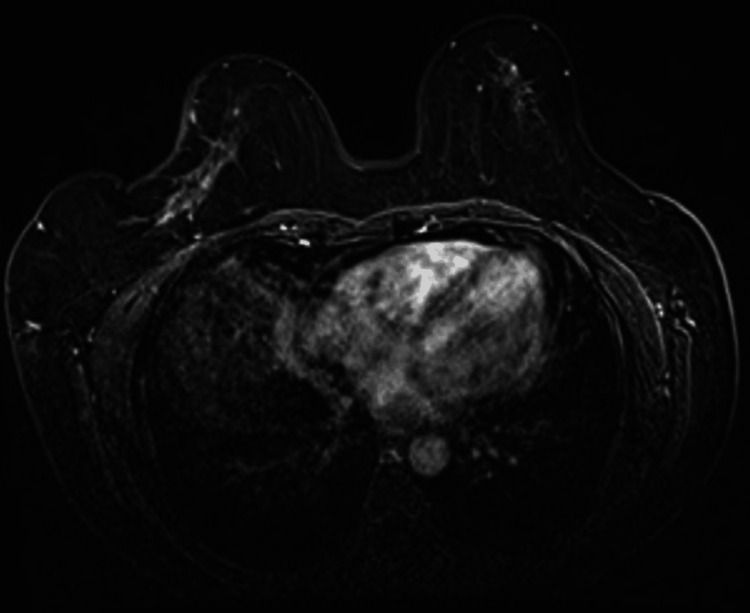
Magnetic resonance mammography (contrast, T1-weighted fat suppressed) showing no lesions in the bilateral breast.
